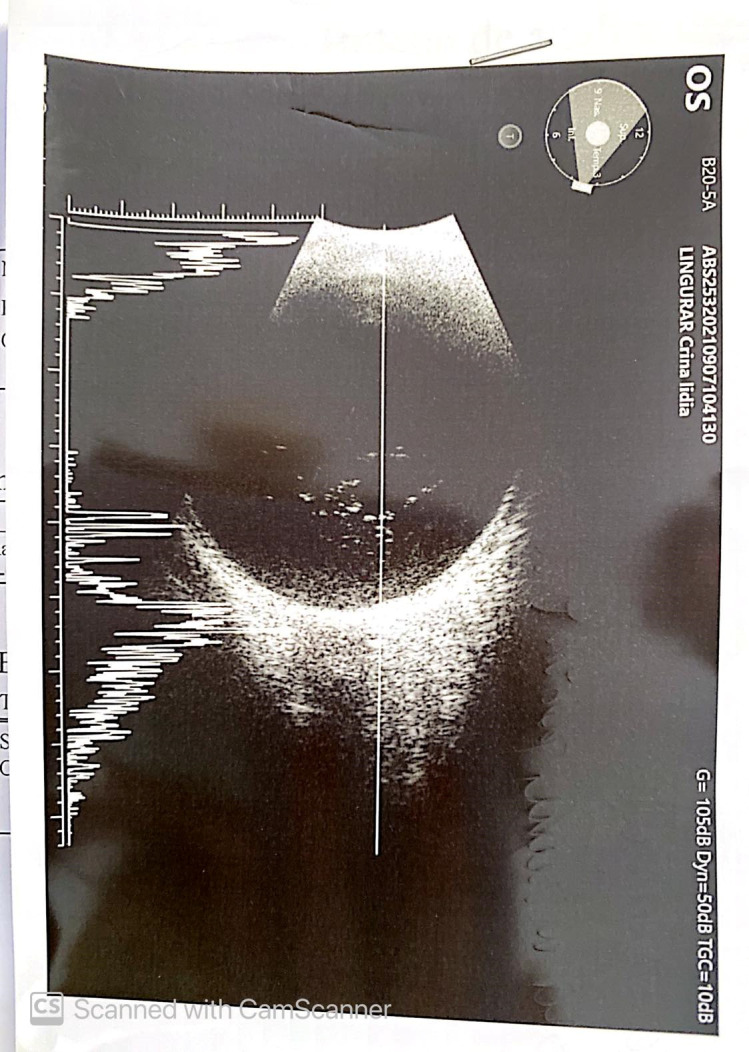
Conventional B-mode ultrasonography - Attached retina, vitreous degenerative echoes
